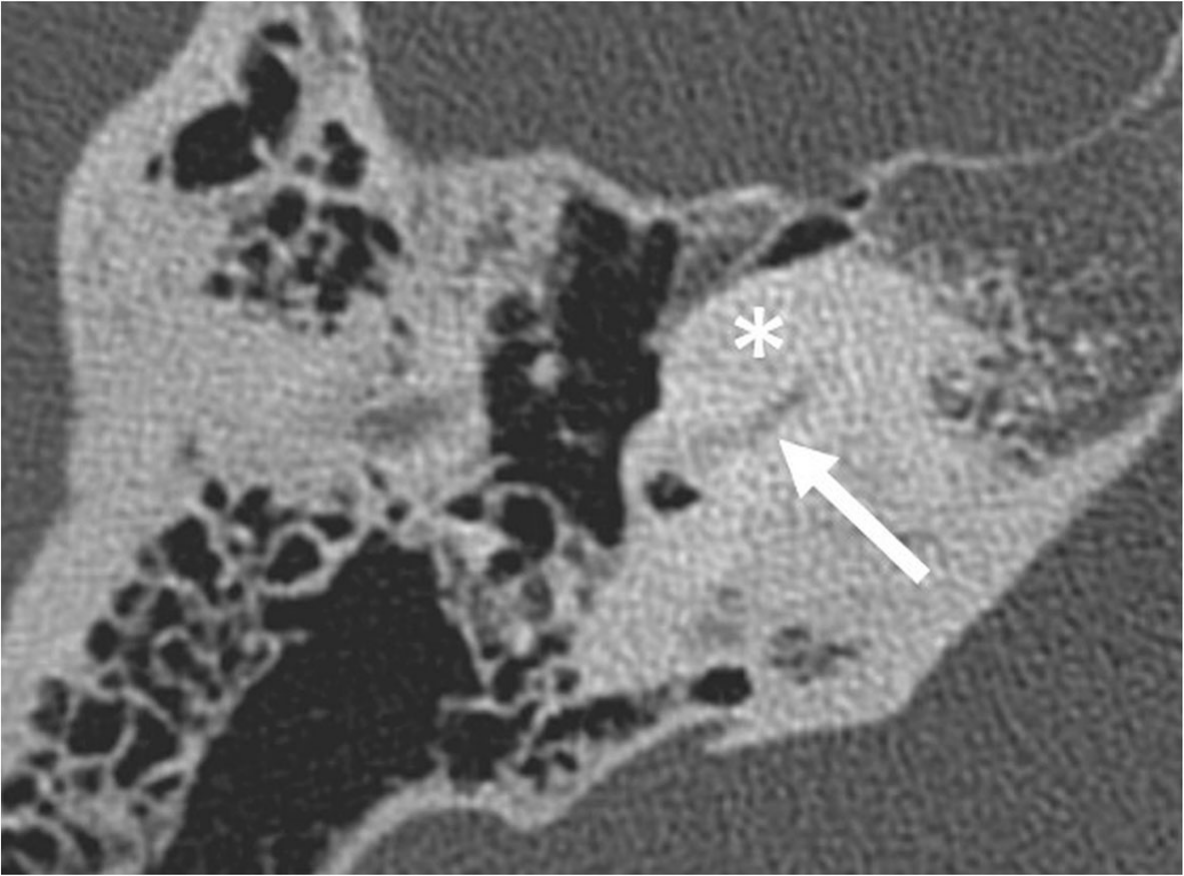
A 38-year-old female patient, with unilateral sensorineural deafness since the age of 12 years. HRCT axial image shows cochlear sclerosis (asterisk). Only a small part of the basal turn of the cochlea can be faintly seen (arrow)
